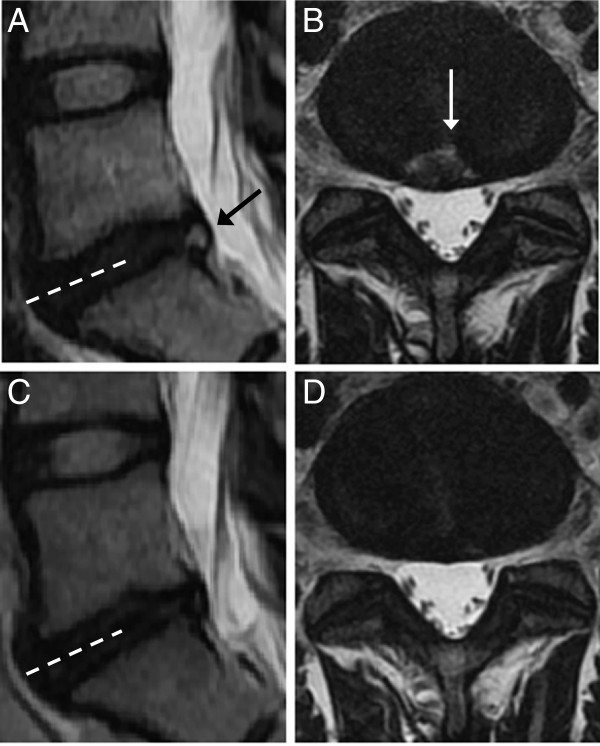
Resolving high intensity zone. Initial (A-B) and follow-up (C-D) T2-weighted magnetic resonance images of one patient. Image plane B is marked on A and image plane D is marked on C (stippled lines). High intensity zone in the L5/S1 disc on initial sagittal (A) and axial (B) images (arrows) is resolved on later sagittal (C) and axial (D) images.
